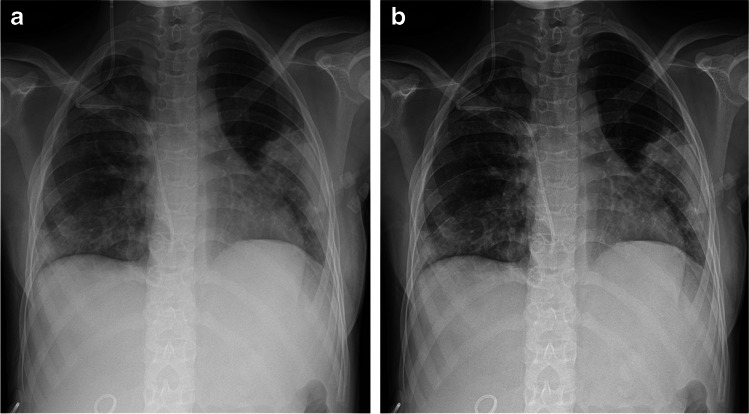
An anteroposterior chest radiograph (69.6 kV, 4.0 mAs, 4.51 cGy·cm^2^, exposure index: 345.50), unprocessed image (a) versus a SimGrid image (b) in an 8-year-old boy (weight: 25 kg) with pneumonia
